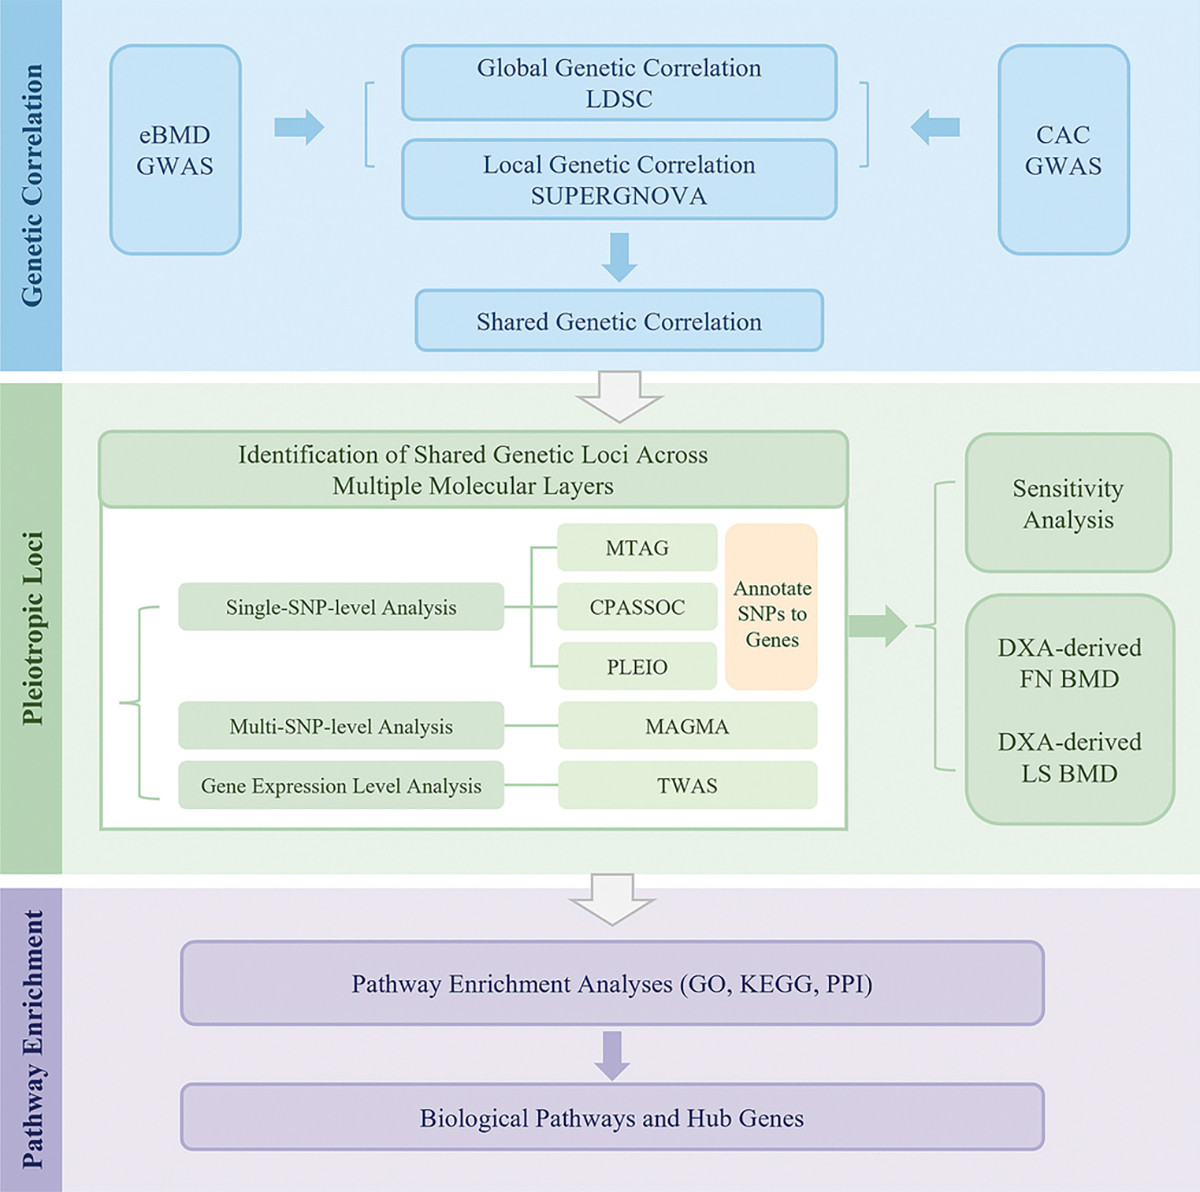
Flow-chart of the overall study design. GWAS: genome-wide association study; CAC: coronary artery calcification; eBMD: estimated calcaneal bone mineral density; LDSC: linkage disequilibrium score regression; MTAG: multi-trait analysis of GWAS; CPASSOC: Cross-Phenotype Association; PLEIO: Pleiotropic Locus Exploration and Interpretation using Optimal test; MAGMA: Multi-marker Analysis of GenoMic Annotation; TWAS: transcriptome-wide association study; DXA: dual-energy X-ray absorptiometry; BMD: bone mineral density; FN: femoral neck; LS: lumbar spine; PPI: protein-protein interaction; GO: Gene Ontology; KEGG: Kyoto Encyclopedia of Genes and Genomes.
